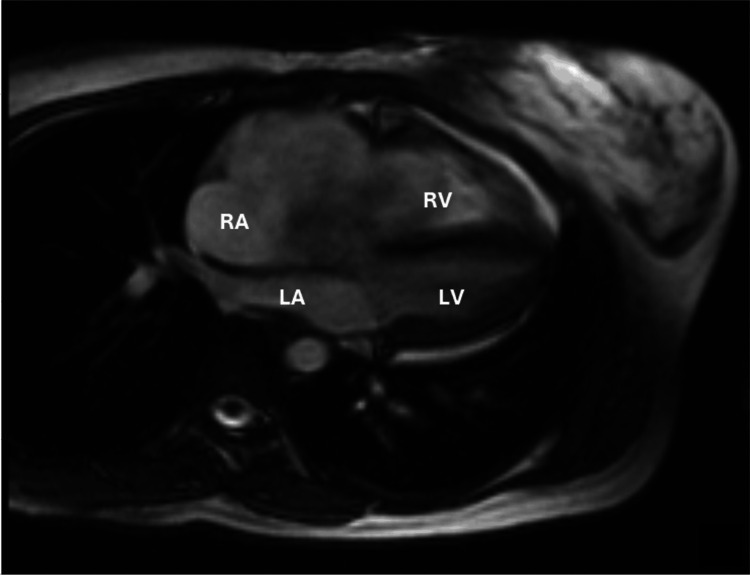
Sagittal cardiac magnetic resonance imaging demonstrating a right atrial myxoma compressing the right ventricle. RA: right atrium; RV: right ventricle; LA: left atrium; LV: left ventricle
